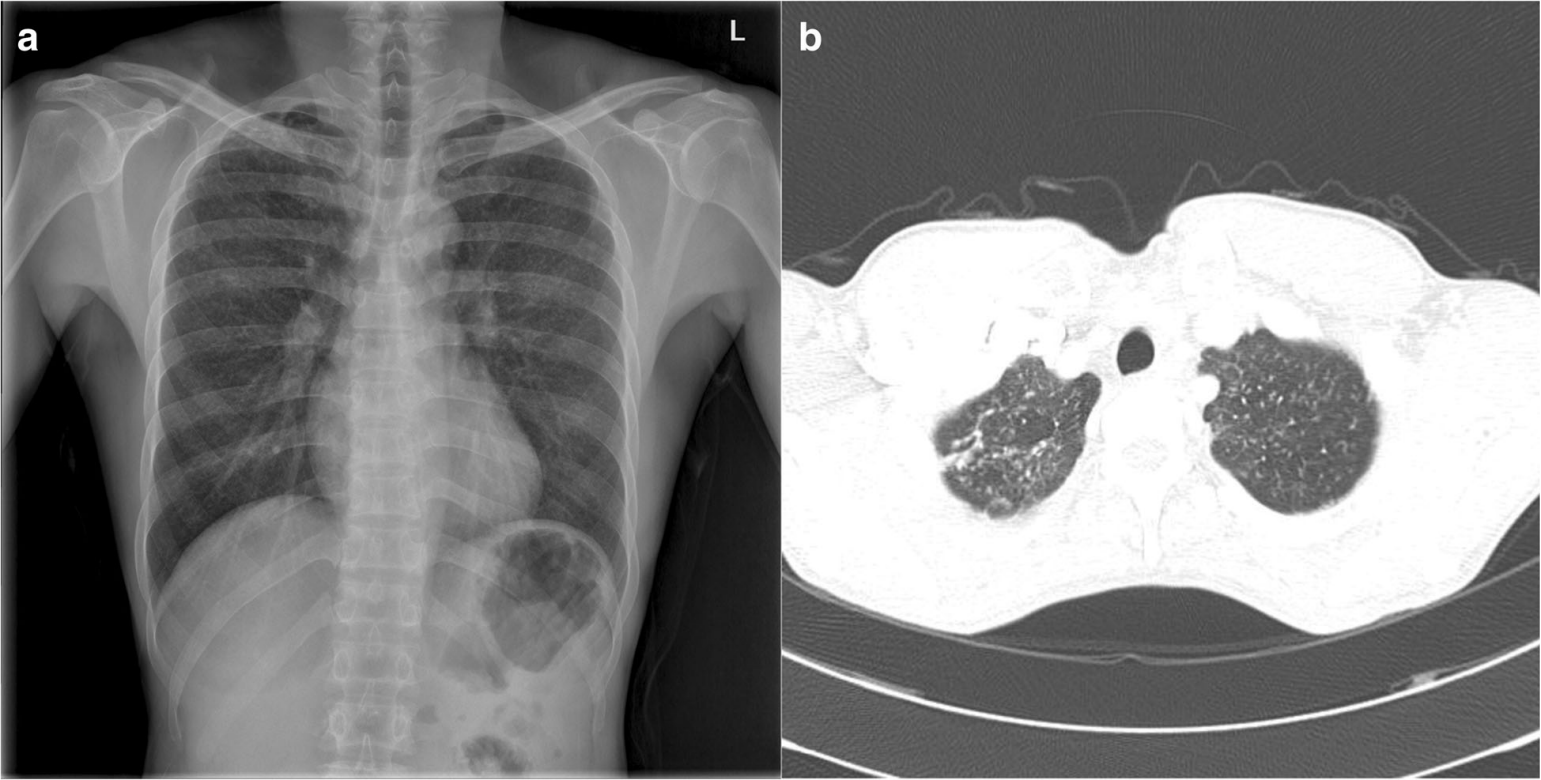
a Chest radiograph showing multiple bilateral nodules in both lung fields. b CT scan of the chest showing numerous tiny nodules with perilymphatic distribution without lymph-node calcification
